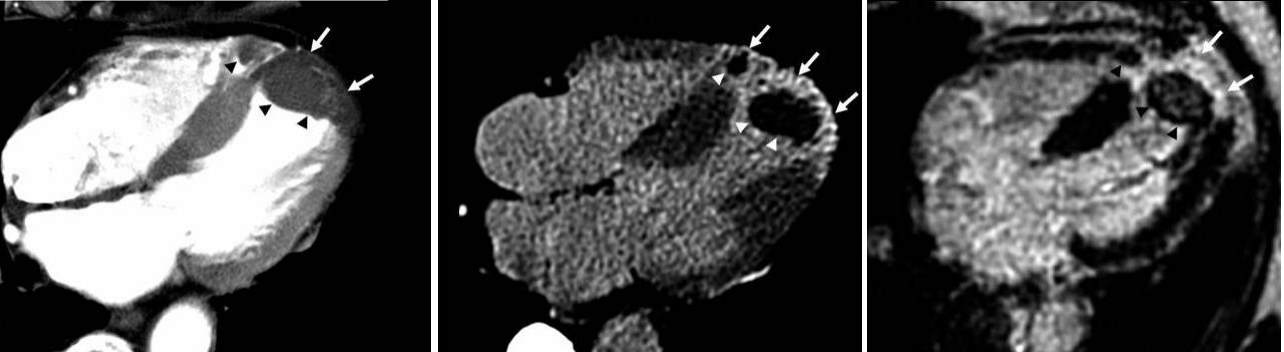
4-chamber view reformat from arterial phase MSCT reveals a perfusion deficit of the left ventricular apex (A). The corresponding late-phase MSCT image presents the delayed myocardial contrast enhancement in the apical-anterior and -septal segments of the left ventricle and the right ventricular apex (B; arrows). Left and right ventricular thrombi are shown (arrowheads). Delayed-enhanced MR image confirms the presence myocardial infarction (arrows) and ventricular thrombi (arrowheads) (C).
